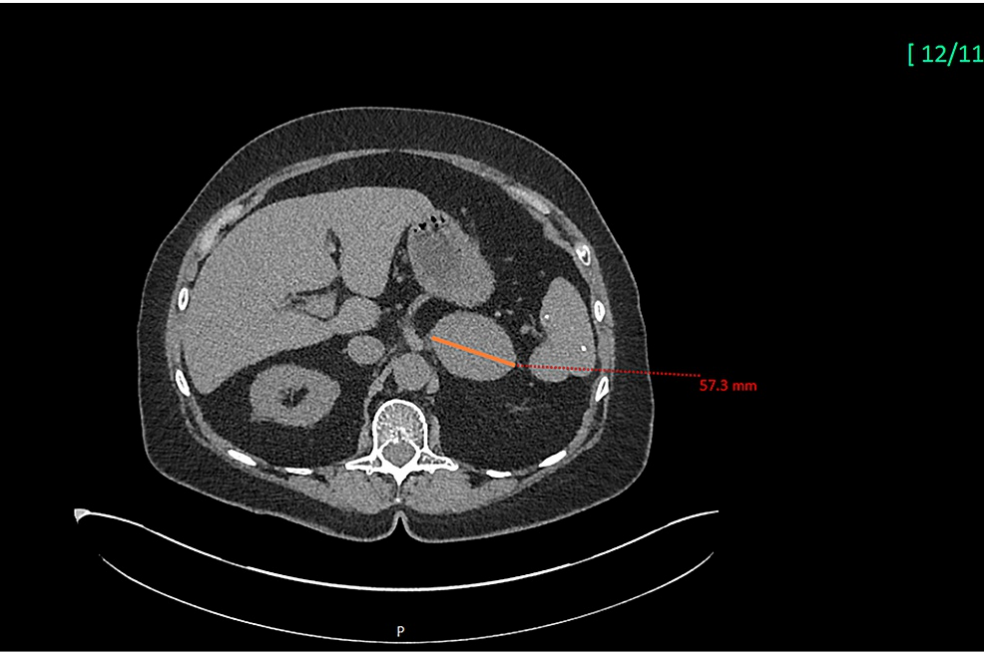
Image showing the progression of the apparently benign left adrenal incidentaloma (arrow in Figure 1) to adrenocortical carcinoma (arrow). CT scan images nine years later showing the change in the characteristics of the lesion, with a rapid interval growth measuring 57.3 mm (maximum diameter). CT: computed tomography
